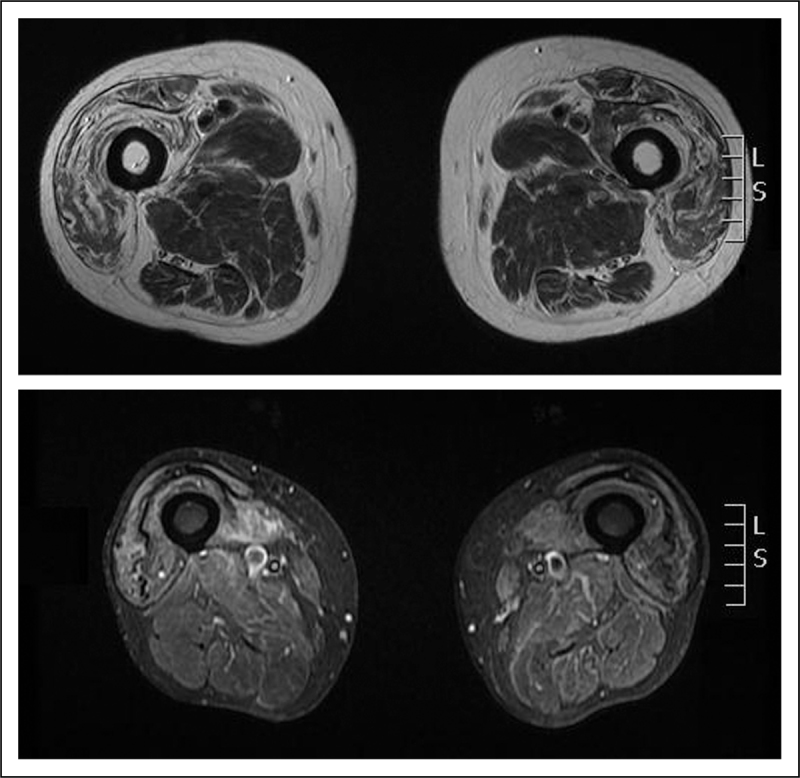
Transverse T1-weighted (upper slice) and STIR image (bottom slice) of the thighs of patients with inclusion body myositis. Upper row: note the fatty infiltration (areas of increased signal) predominantly of the anterior muscles of the thigh. Bottom row: note the areas of high signal in the right thigh (also in the anterior muscles), indicating muscle oedema (inflammation).
